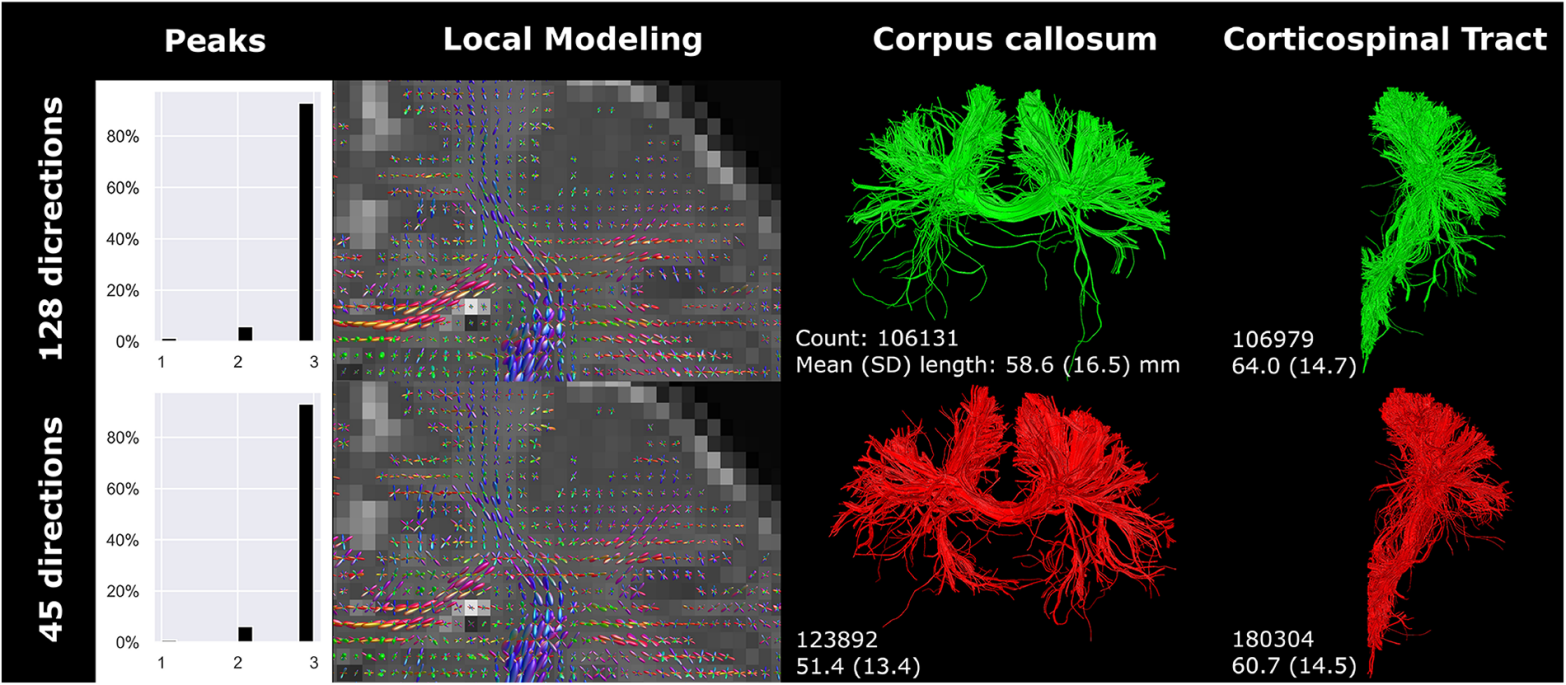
High-quality diffusion MRI data from the developing human connectome project was down sampled from 128 (single-shell; upper row) to 45 (lower row) gradient directions. Branching/fanning of the corpus callosum was visibly affected by down sampling. Reconstructions were acquired from the dHCP scan.
